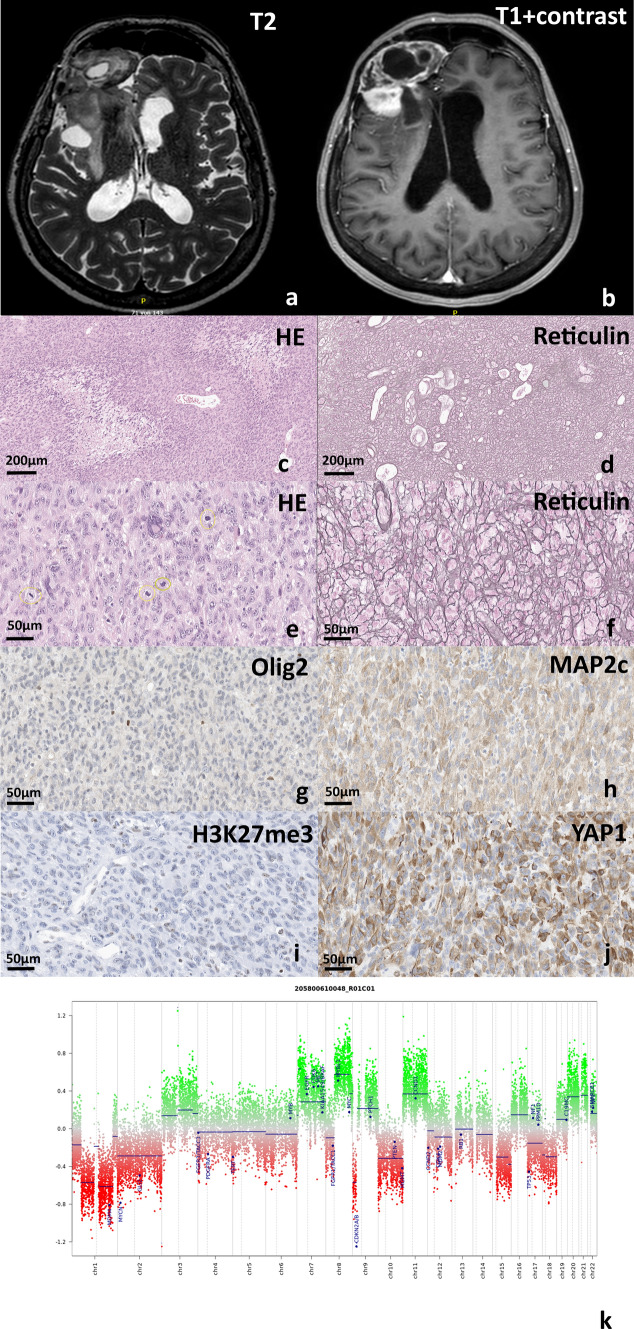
MRI showed a contrast-enhancing multi-lobulated and cystic appearing tumor within the previous resection cavity (a, b). The tumor revealed a diffuse sarcomatous histo-morphology with areas of palisading necrosis (c) and endothelial proliferations (d), many mitotic figures (e), abundant reticulin (f), lack of Olig2 expression (g) but expression of MAP2c (h), reduced H3K27me3 expression (i), and extensive expression of oncogenic YAP1 (j). Copy number profile of the tumor revealed multiple chromosomal alterations also including typical constellation + 7/−10 and homozygous deletion of *CDKN2A/B* usually present in glioblastoma/ gliosarcoma, IDH-wildtype (k)
